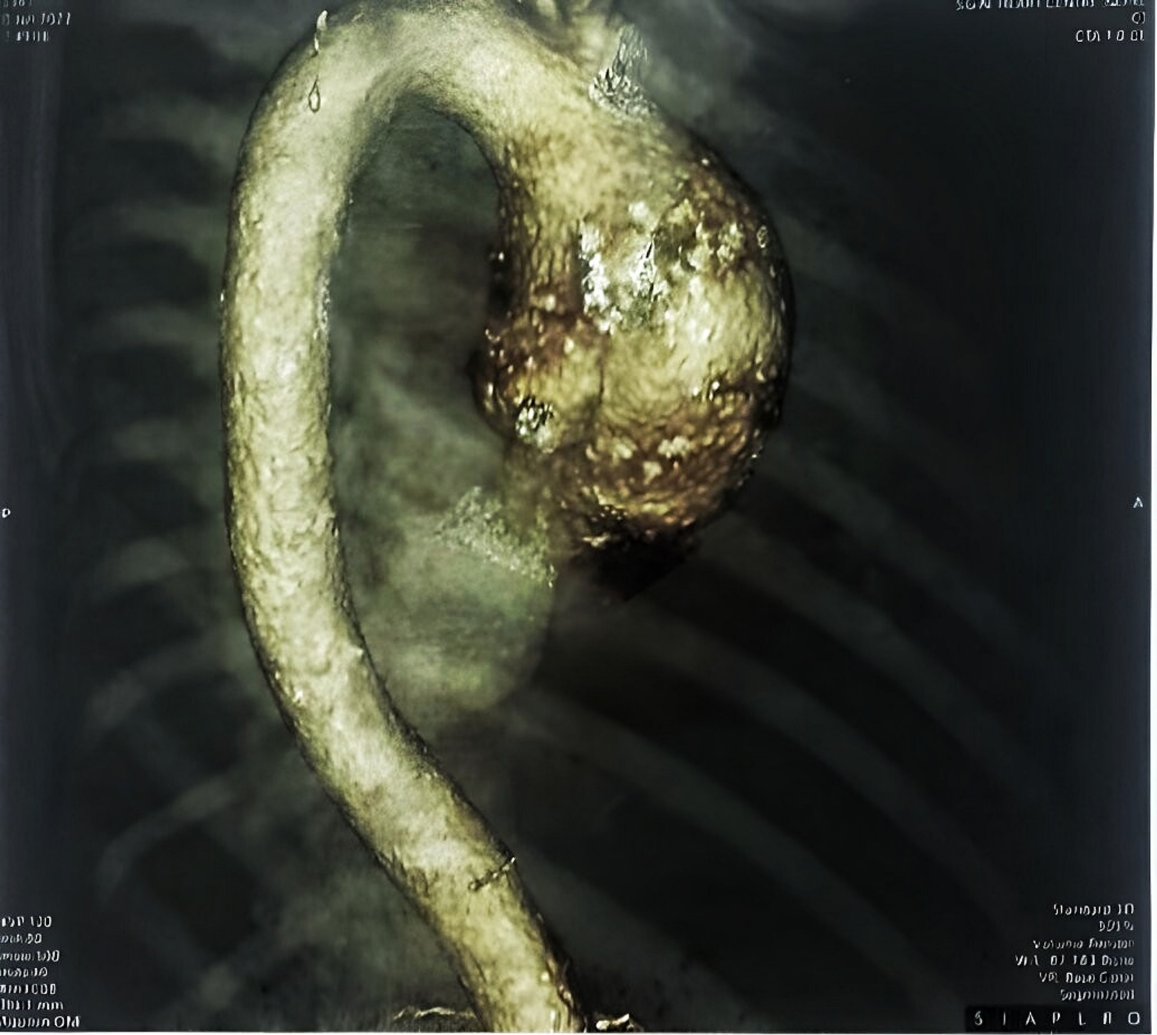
3D reconstruction of the aorta from the CT angiogram of the patient showing an ascending aortic aneurysm.
